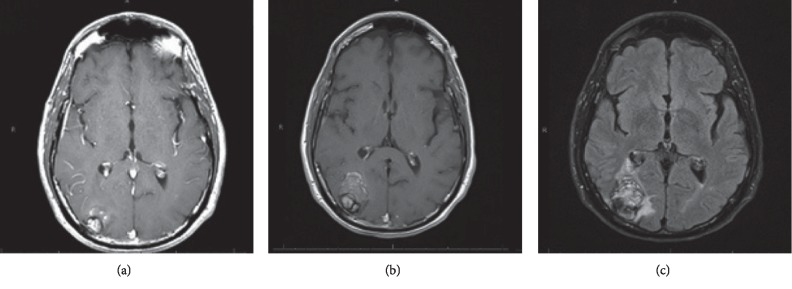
(a) Axial view T1 with Gadolinium at presentation, showing right posterior temporal lesion with a T1 hypointense rim suggestive of atypical stroke. Images of new findings at the previous area of stroke to evaluated new neurologic symptoms: (b) axial view T1 with Gadolinium showing an internal increase in size and (c) axial view FLAIR showing a heterogeneous lesion with hypointense foci within associated surrounding edema and enfacement of posterior horn or right lateral ventricle.
